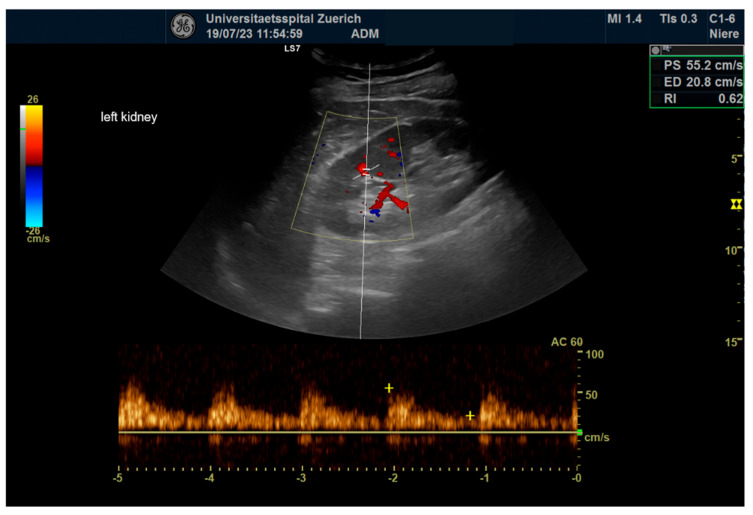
Color coded duplex sonography of renal resistance index values in renal ultrasound.
